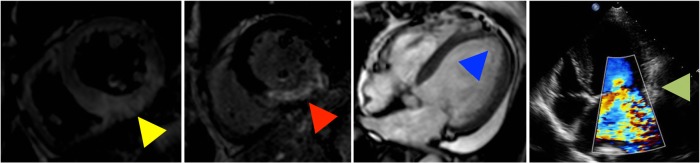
Examples for CMR findings of myocardial edema, necrosis, cardiac remodeling and echocardiography finding of severe mitral regurgitation (from left to right). The absence of detectable myocardial necrosis (red arrow head) is a key criterion for Grade-0 patients, for whom, myocardial edema (yellow arrow head) can be the only finding in the acute phase. Cardiac remodeling is defined as an expanded left ventricle (blue arrow head). Apparent mitral regurgitation (green arrow head) on the basis of cardiac remodeling is indicative of Grade 4.
